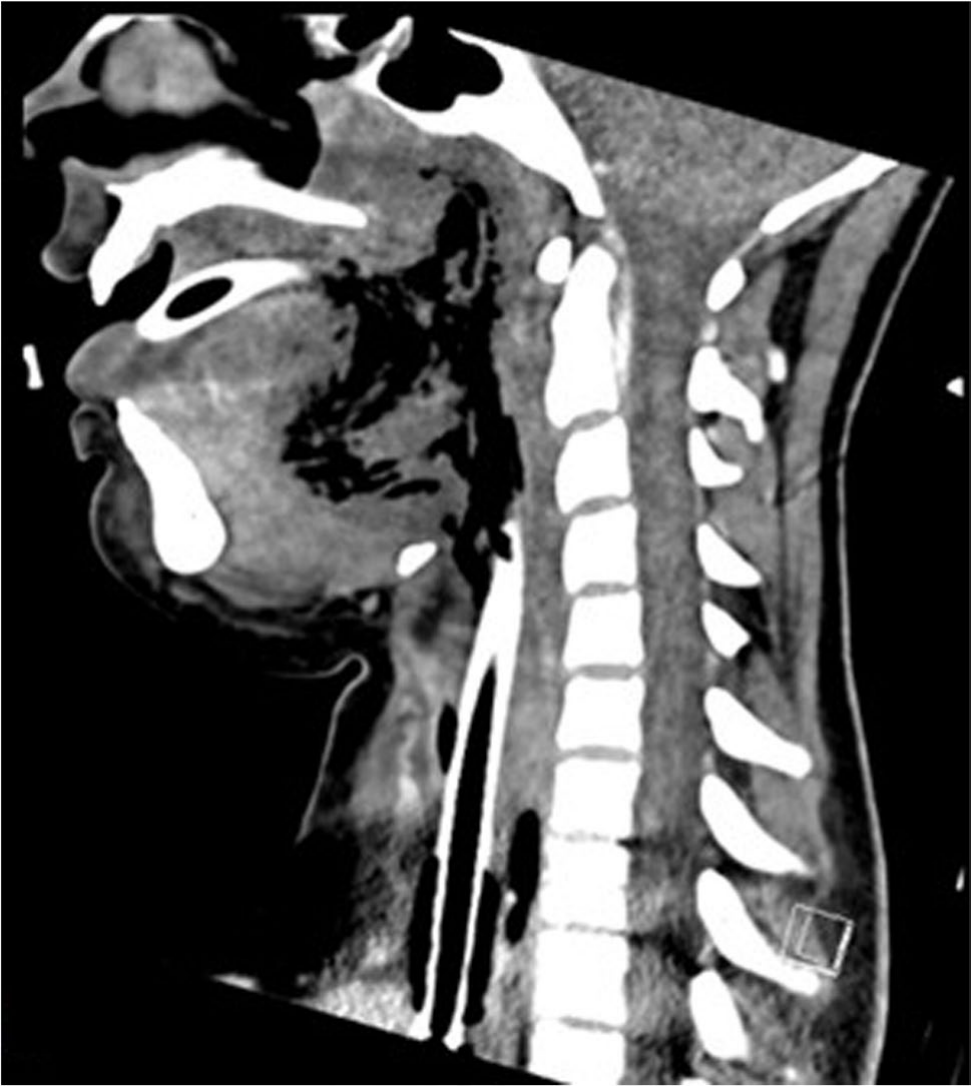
Contrast-enhanced CT scan revealing deep neck soft-tissue inflammation with necrosis extending toward the carotid spaces
